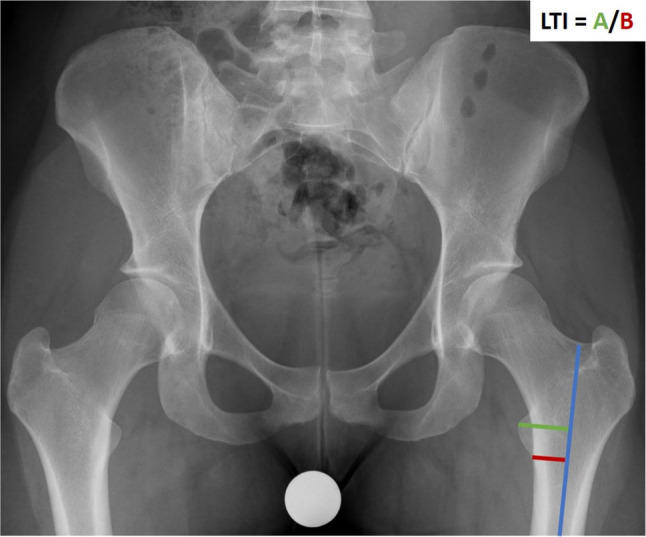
Representative case to calculate the LTI on pelvic radiographs
